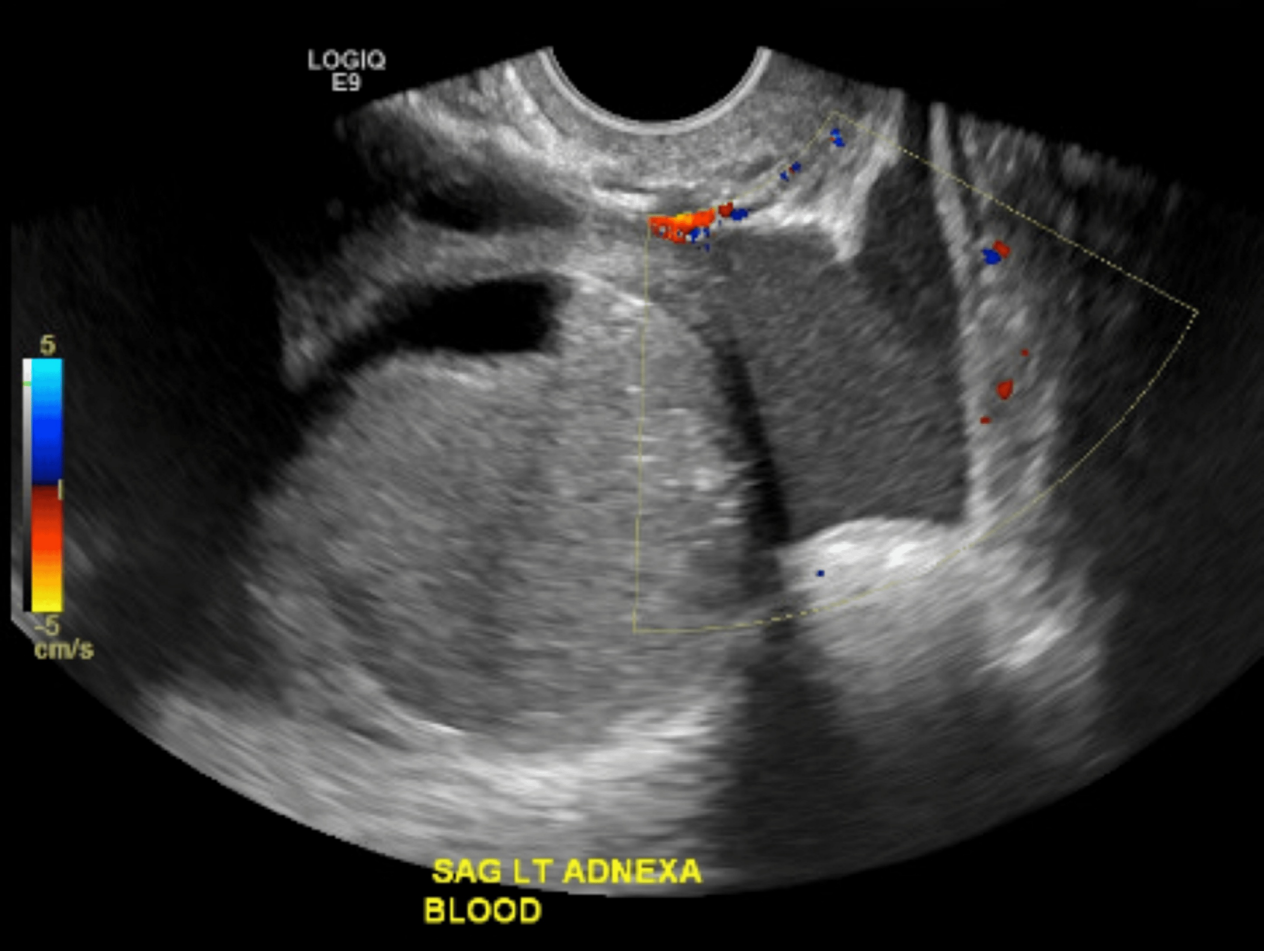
Ultrasound sagittal view of the left adnexa and blood This ultrasound image shows a different viewpoint of the left adnexa, including the left-sided ovary and fallopian tube. Also shown here is the presence of blood and blood flow.
